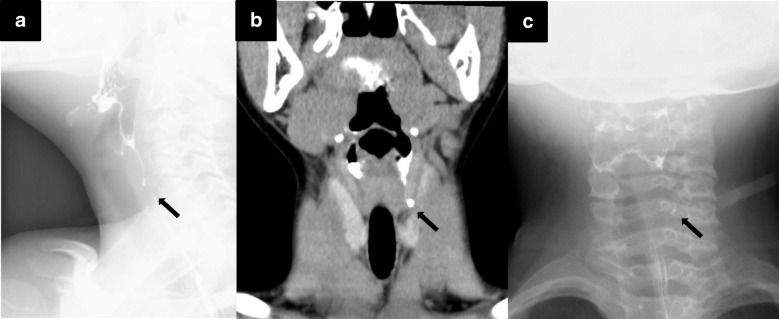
a Barium esophagography shows a pyriform sinus fistula (arrow) on the left side. b Oral contrast coronal computed tomography shows a fistula (arrow). c Barium esophagography after open neck surgery shows recurrence of the pyriform sinus fistula (arrow)
